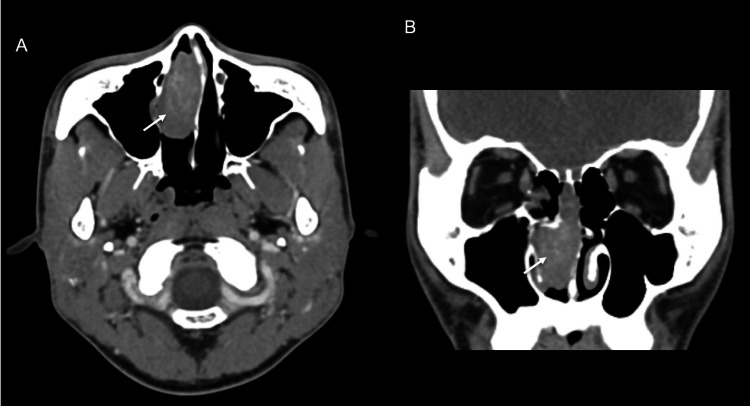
CT depicted a tumor in the right nasal cavity (arrow) causing contralateral bowing of the nasal septum with the erosion of the nasal septum and right lateral nasal wall. (A) Axial cut showed anteroposterior extension of the tumor whereas (B) coronal cut showed superior extension towards the roof of the nasal cavity.
